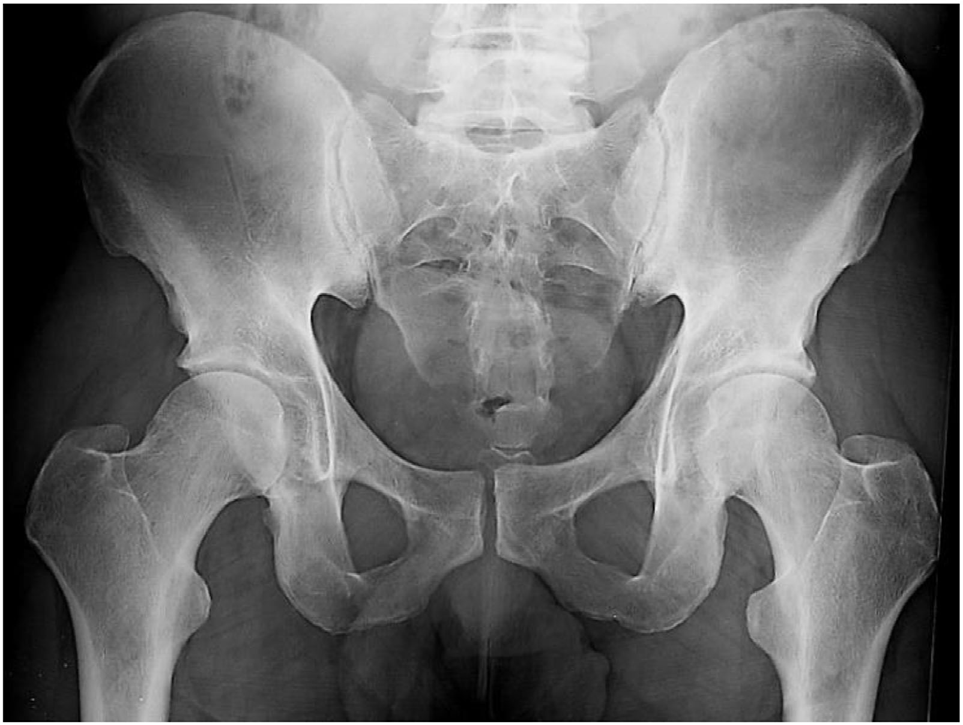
A 40-year-old male with a 3-year history of low back pain with short duration morning stiffness. Treatment with nonsteroidal anti-inflammatory drugs showed partial improvement. B27 is negative and C-reactive protein is 20 mg/L. Pelvic radiograph demonstrates suspicious changes of the right sacroiliac joint (SIJ) and minimal abnormalities of the left SIJ, not fulfilling the 1984 modified New York criteria for the diagnosis of ankylosing spondylitis.
